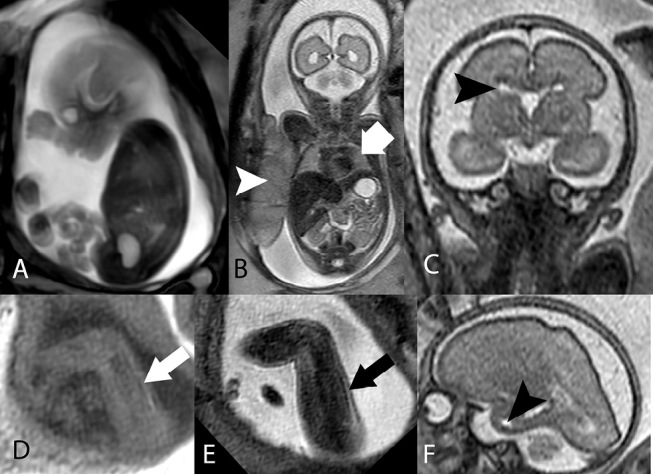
MR Phenotyping in a case of native american myopathy (STAC3 gene mutation, OMIM: 615521): Dynamic T2-weighted sequence of a Fetus at 28+1 GW (A), indicating bilateral talipes and camptodactyly (bent fingers); on a coronal T2-weighted sequence (B) an abnormally lobulated placenta (white arrowhead) and small lungs (white bold arrow) are visible. Coronal (C) and sagittal (F) T2-weighted images of the fetal brain show enlarged outer cerebrospinal fluid spaces as well as periventricular cysts (dark arrowheads). Sagittal T1 weighted (D) and T2-weighted sequences indicate abnormal skeletal muscle signals (T1 bright and T2 bright) indicating fatty replacement and the presence of a myopathy.
